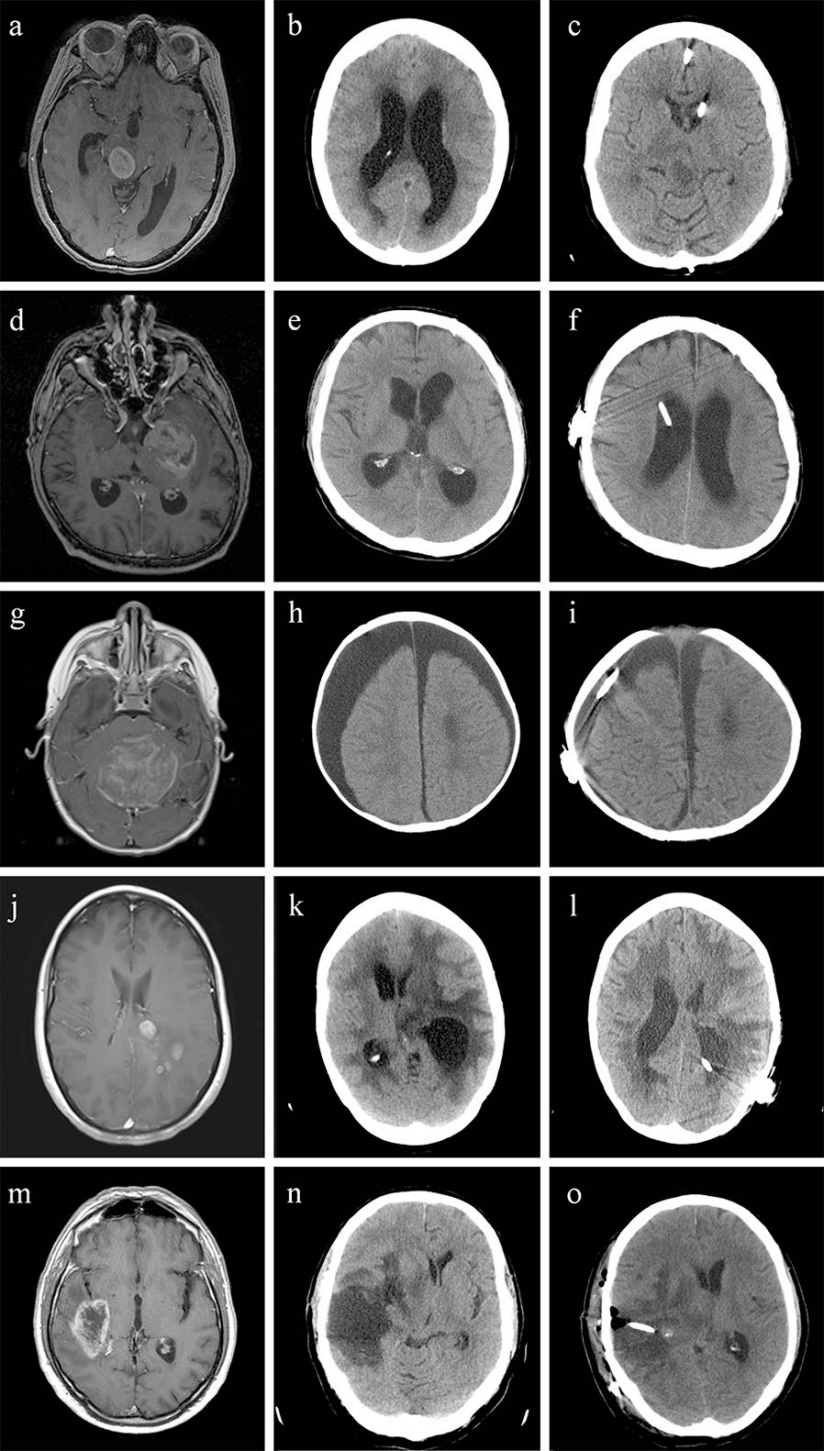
Images of glioblastoma patients: left column T1-weighted MR image after administration of gadolinium, centre collumn native CT scan showing CSF circulation disturbances and right collumn postoperative native CT scan after shunt placement. a A 41-year-old woman shows a pontomesencephalic glioblastoma in with compression of the aqueduct. b Widening of the lateral ventricles due to obstructive hydrocephalus. c Reduction of ventricular size after implantation of a ventriculoperitoneal shunt. d A 64-year-old man shows a glioblastoma in the left temporomesial lobe. e Ventricular enlargement due to communicating hydrocephalus. f The intracranial catheter in situ after implantation of a ventriculoperitoneal shunt. g A 1-year-old boy shows a midline glioblastoma, which resulted initially in obstructive hydrocephalus. h External hydrocephalus with extensive subdural hygroma. i After implantation of a subduroperitoneal shunt. j A 43-year-old woman shows a glioblastoma in the left parietal lobe/subcortical white matter. k Three weeks after tumor resection, CT imaging reveals isolated extension of the left posterior horn with local compression of adjacent structures and midline shift. l A shunt catheter in the posterior horn after implantation of a ventriculoperitoneal shunt. m A 69-year-old man shows a glioblastoma in the left temporal lobe. n A space occupying fluid collection in the resection cavity. o The proximal shunt catheter inserted in the resection
